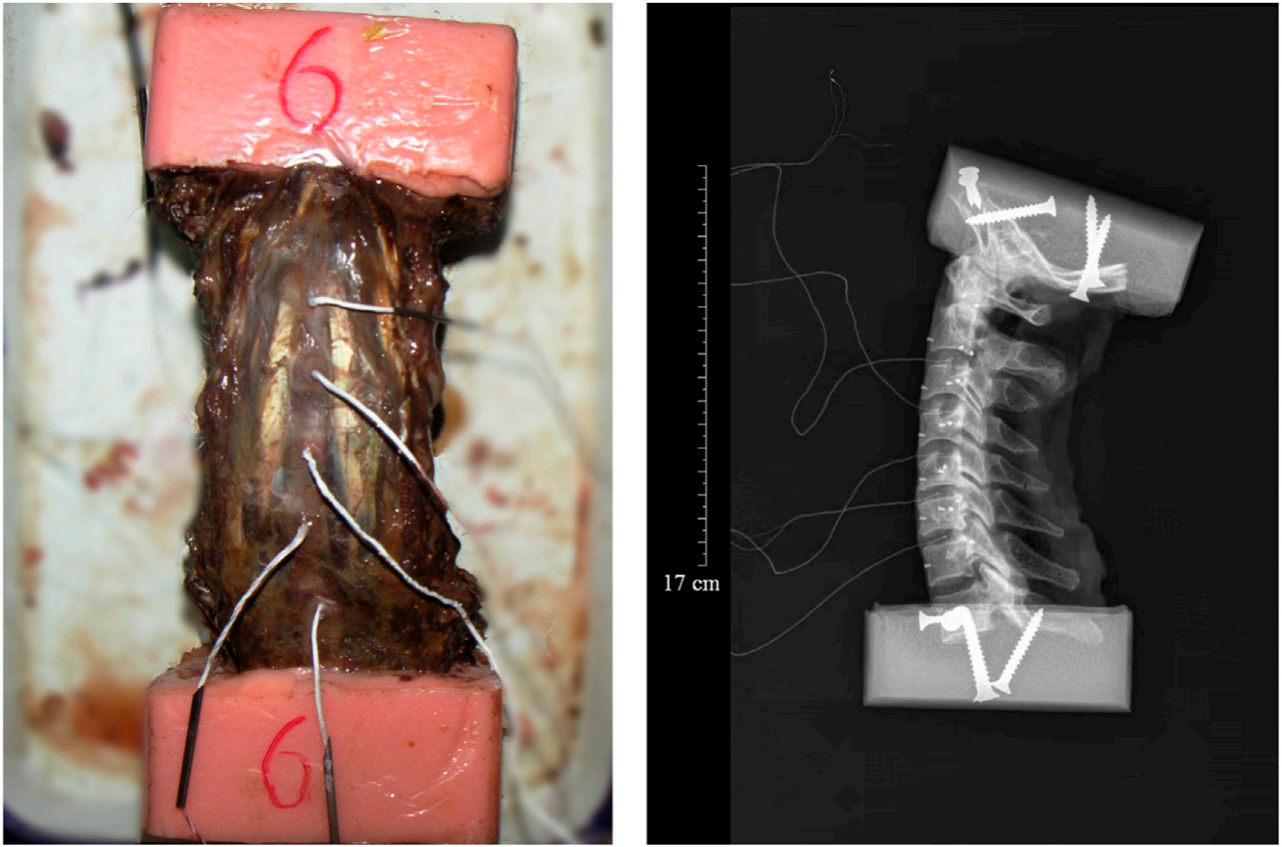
Cervical spine spicemen with the Miniature pressure sensor embedded in the nucleus pulposus (Left) and X-Ray of cervical spine spicemen (Right).
